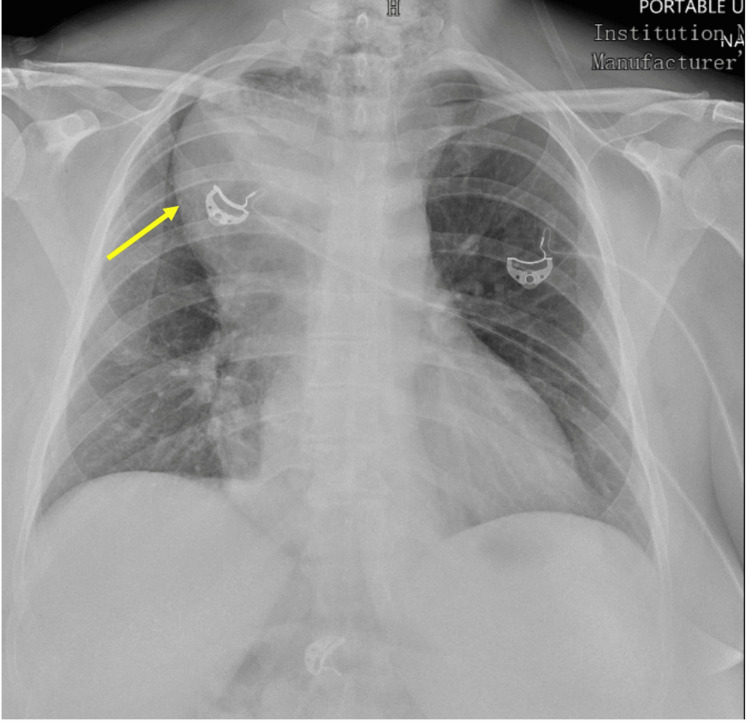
Chest X-ray on admission depicting broadening of the mediastinum
